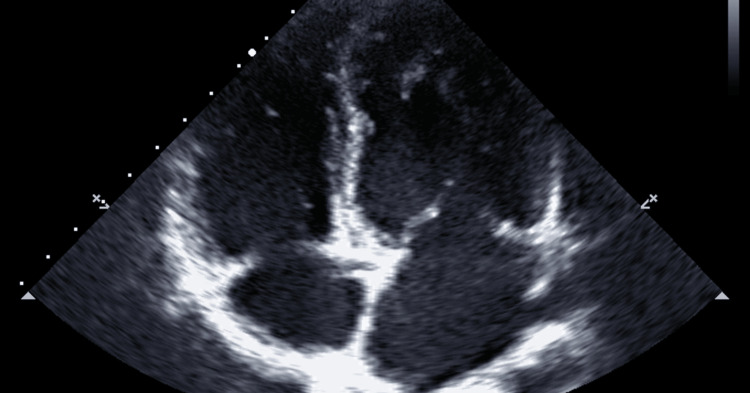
Case 2: Echocardiographic view showing dilated cardiomyopathy with marked left ventricular enlargement and a reduced ejection fraction of 35-40%.
